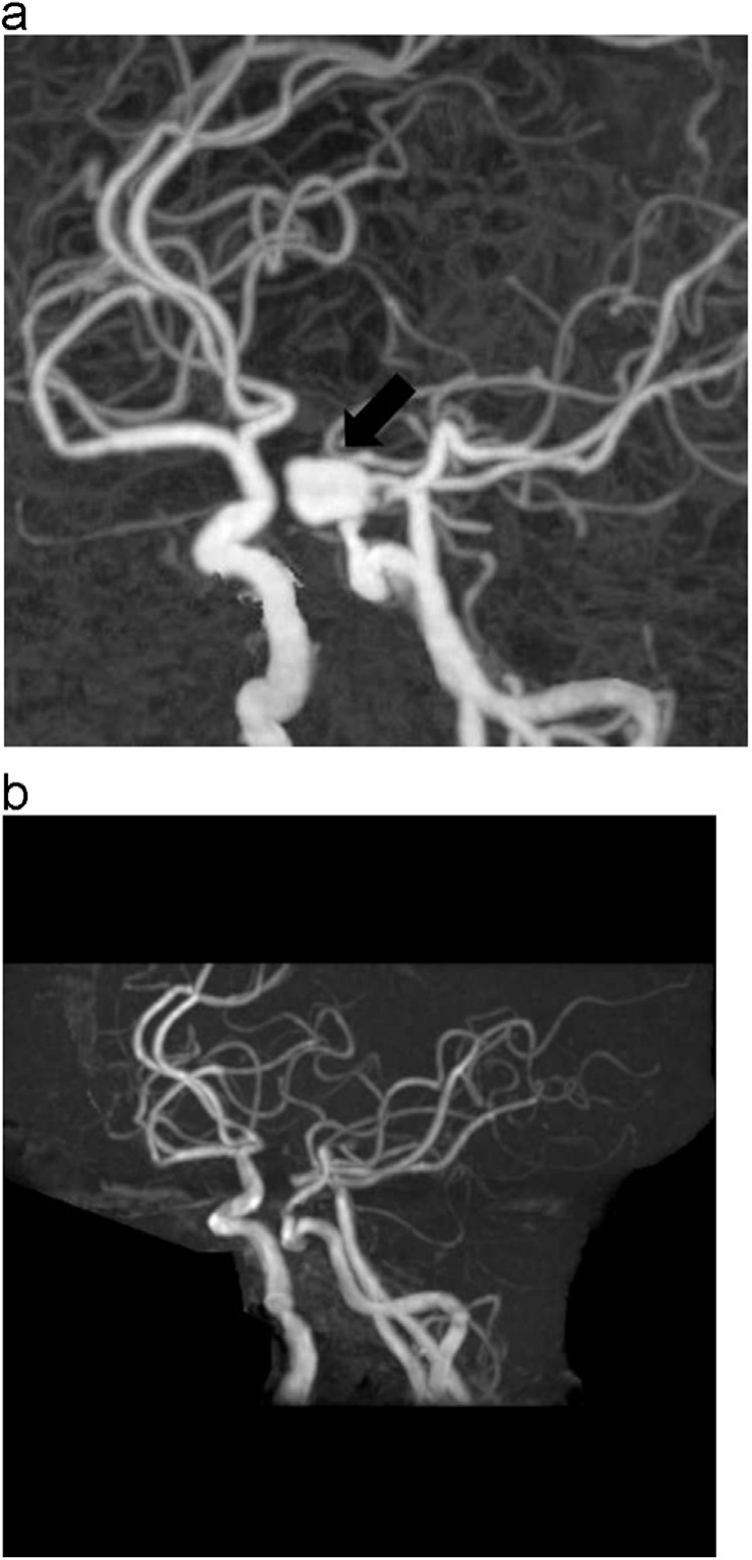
A. CTA revealed an intracranial aneurysm (14×14×15 mm) in proximity to the left sphenoid sinus (➡). B. The aneurysm was absent on the MRA two weeks previous.
